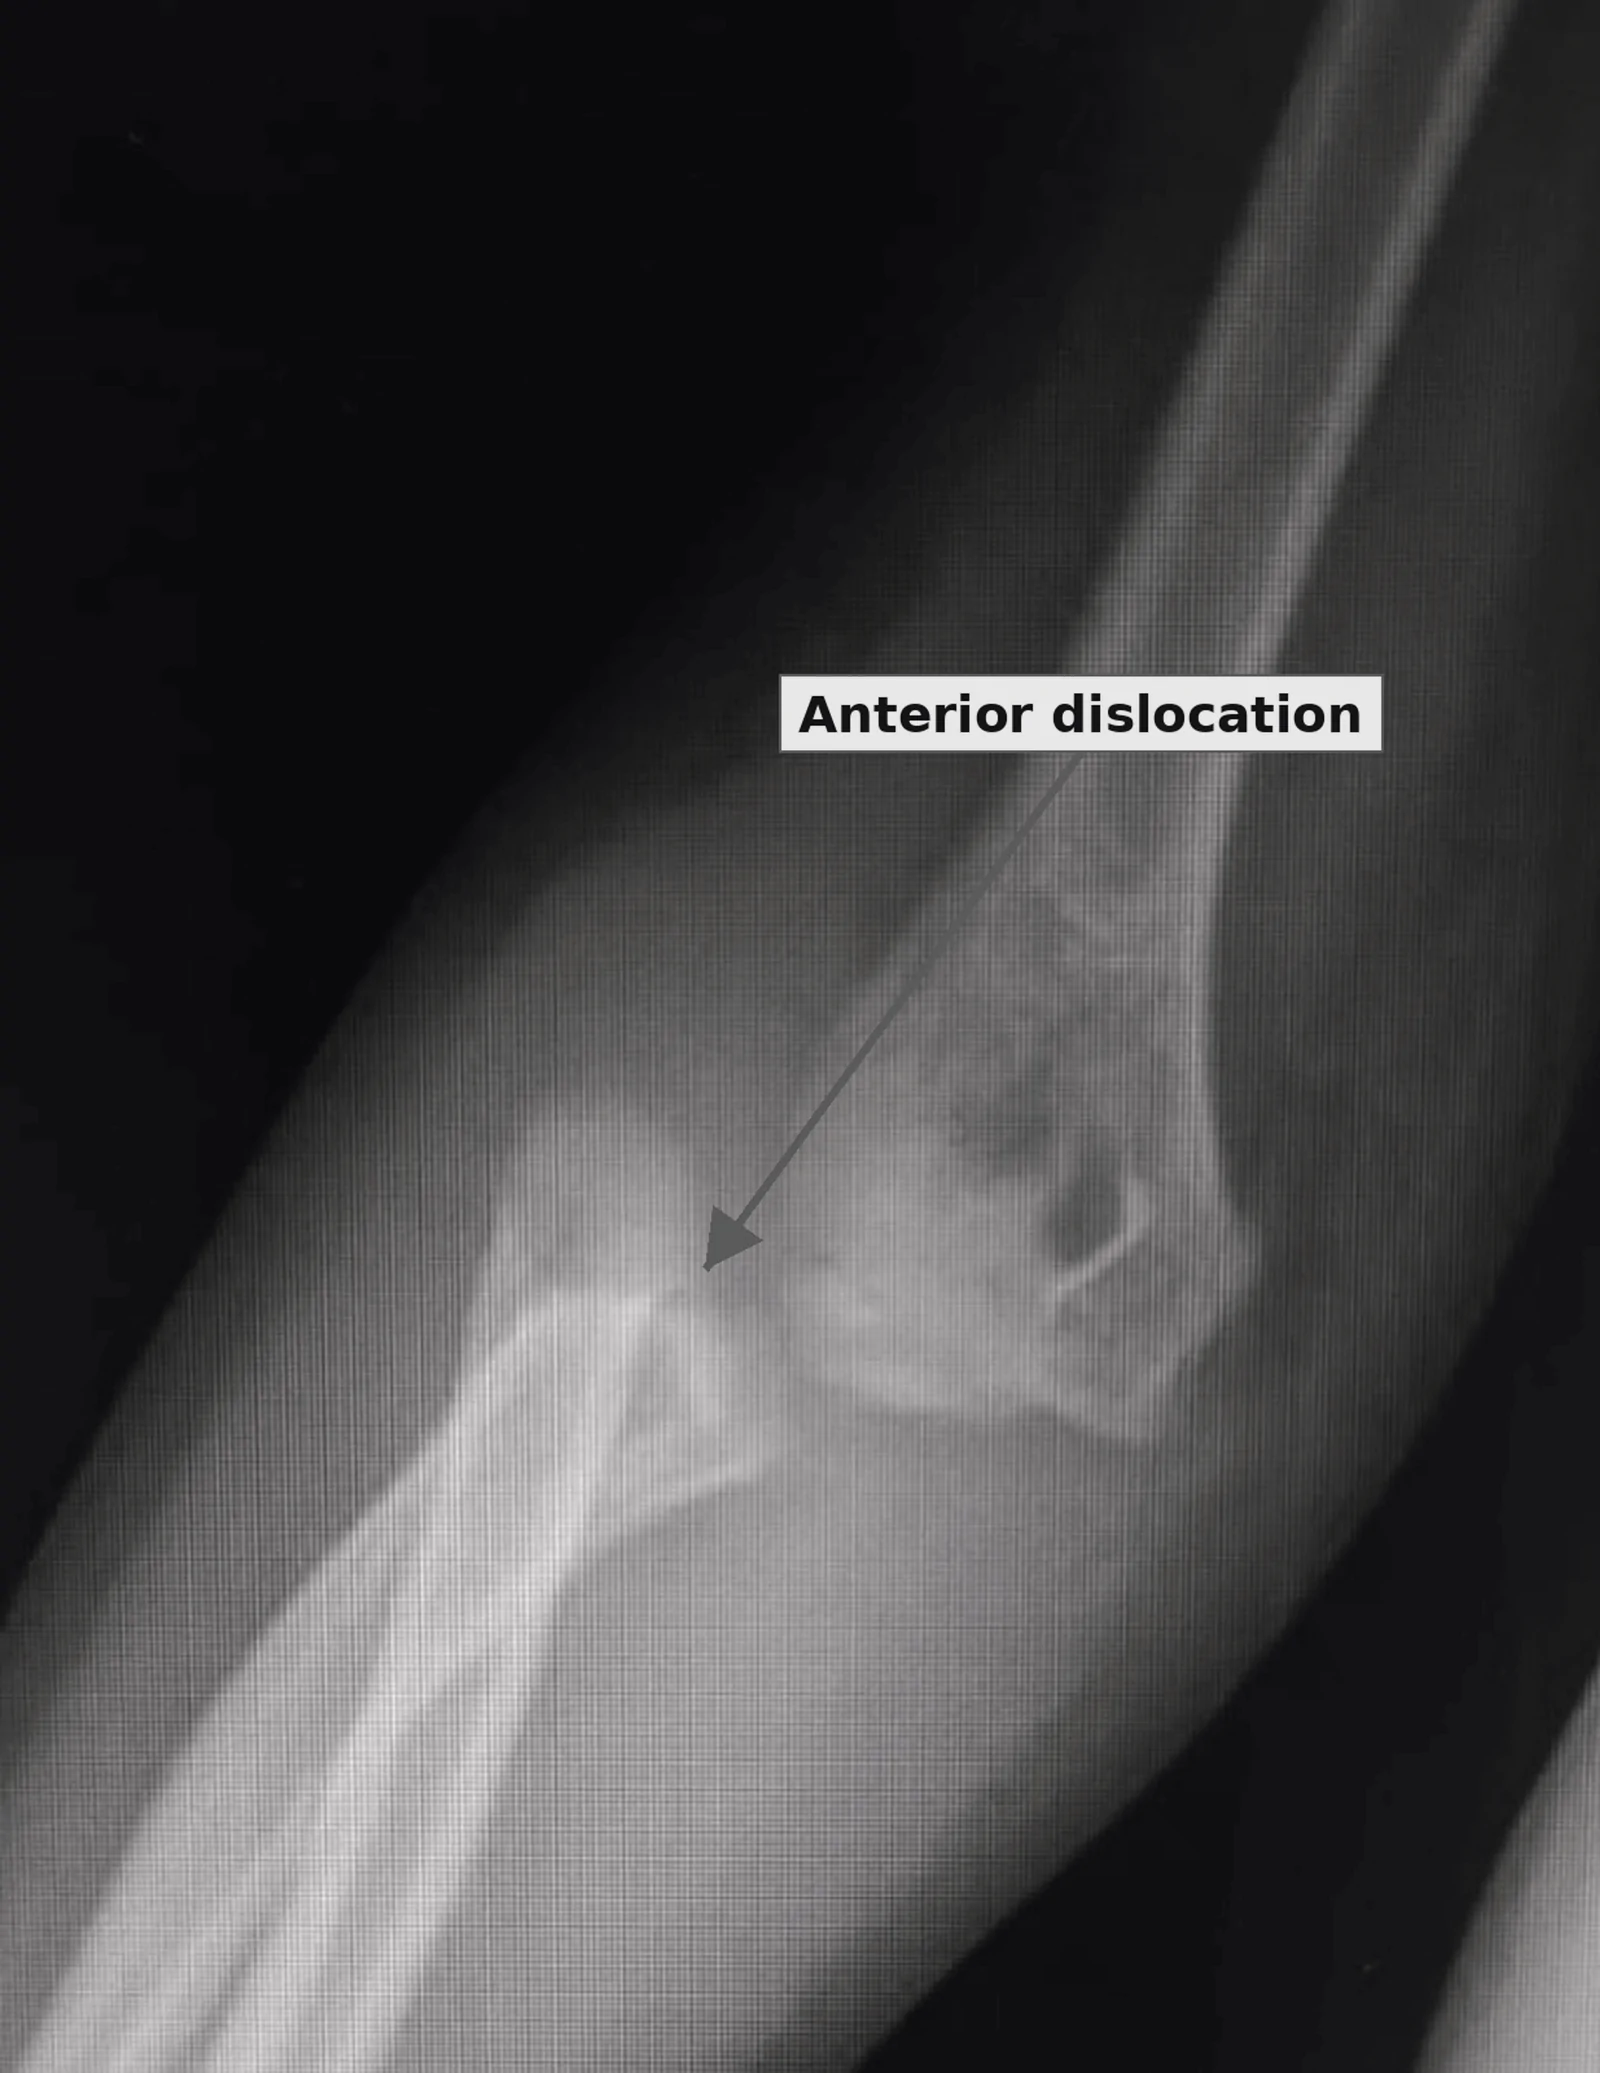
Anteroposterior radiograph of the right elbow confirming anterior dislocation with apparent associated osseous fragments involving the medial epicondyle and humeral condyle prior to reduction.
